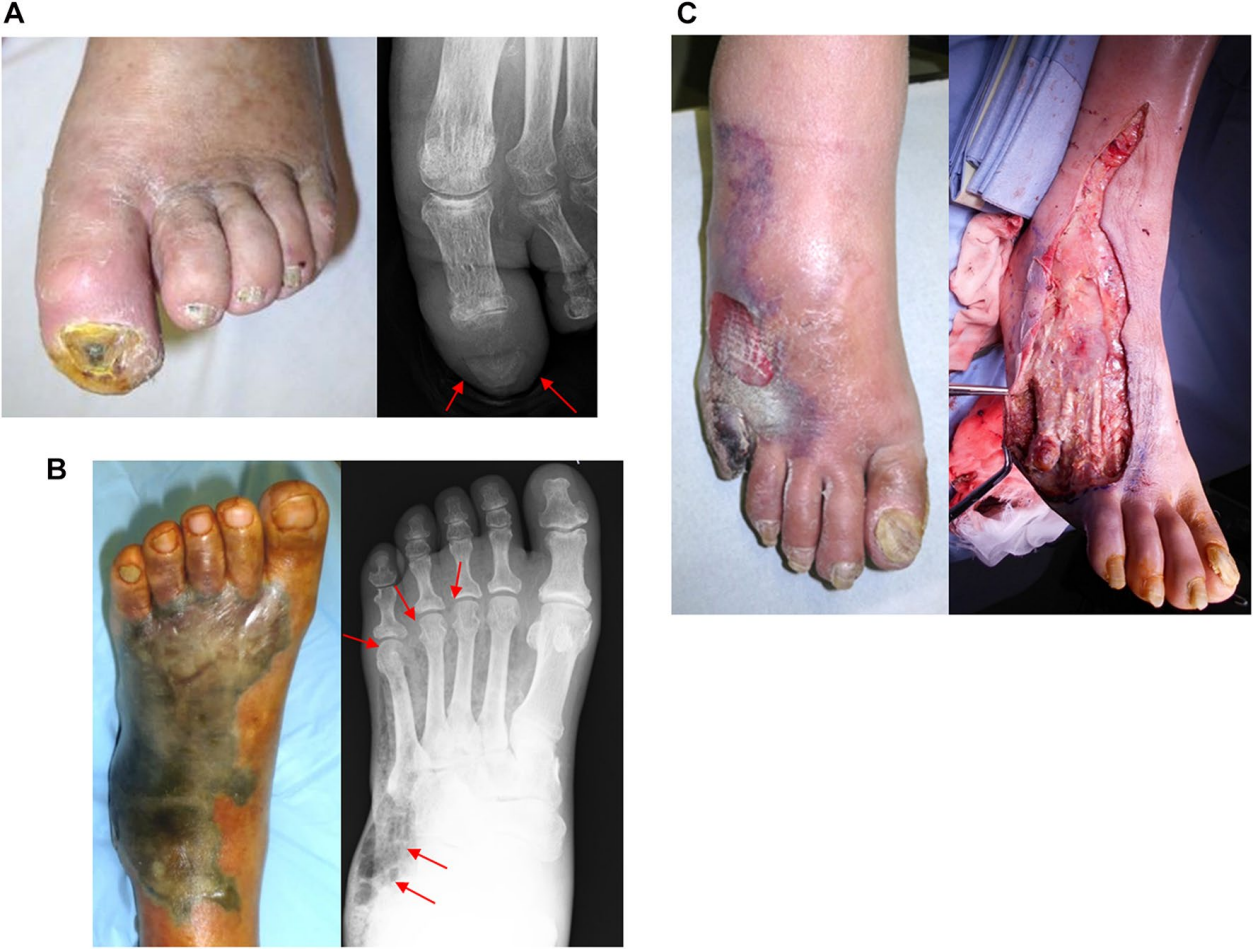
Potential for underlying severe infectious disease in patients with critical limb ischemia. a Osteomyelitis. Only a tiny necrotic lesion was visible on the top of the great toe. However, radiography demonstrated osteolysis of the distal phalanx, suggesting osteomyelitis. b Gas gangrene. Extensive gangrene on the dorsum of the foot was visible. Physical examination revealed snowball crepitus and radiography demonstrated the presence of air in the foot, suggesting gas gangrene. c Necrotizing fasciitis. Gangrene on the lateral side of foot with relative sparing of the skin was visible. Given the rapidly progressive course, severe inflammation, and high CRP value, necrotizing fasciitis was suspected. Emergent surgical incision demonstrated extensive necrosis of the subcutaneous fat and fascia with abscess formation
